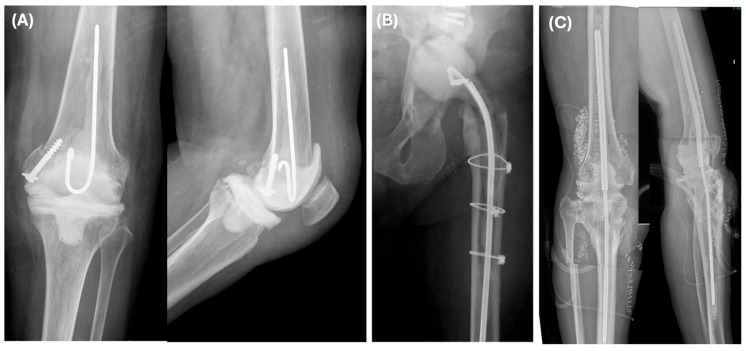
Radiographic examples following the first stage of revision surgery: articulated knee spacer (A), articulated hip spacer (B), and static knee spacer (C) used in a case of extensive bone loss.
